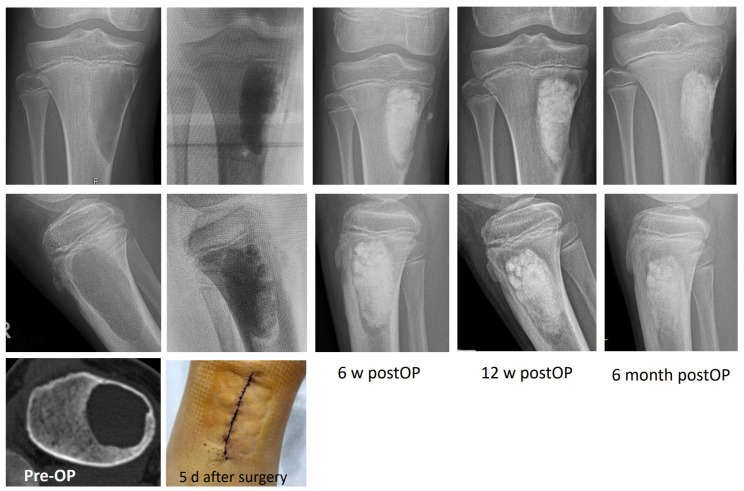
Radiographic 6-month follow-up of a 11-year-old male patient with clinically apparent solitary bone cyst. Due to the fracture risk by eggshell-thin lateral cortical bone and the performance of contact sports, the cyst was curetted by a cortical window and treated by activated TCP granules. Follow-up showed an uneventful wound and complete bone healing, including remodeling of the cortical bone and partial resorption of the biomaterial. The latest clinical follow-up 1 year after surgery showed a fully active and pain-free patient.
